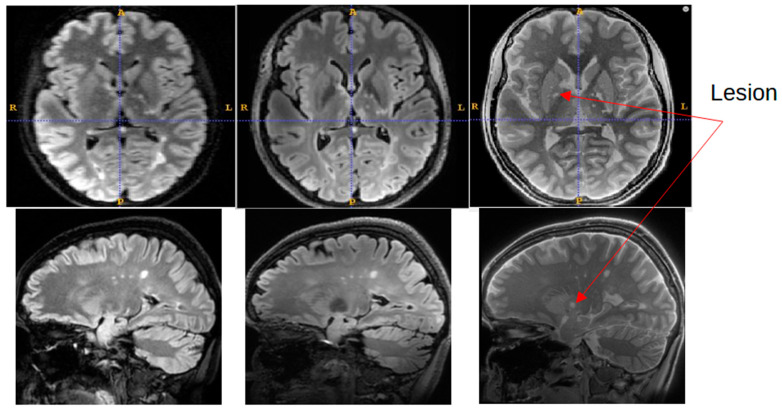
Lesion detected on SilentMT (red arrows) but not observed on axial 1.5T FLAIR or 7T FLAIR. Subsequent radiologic review showed the lesion with a faint and blurred appearance on 1.5T sagittal FLAIR.
